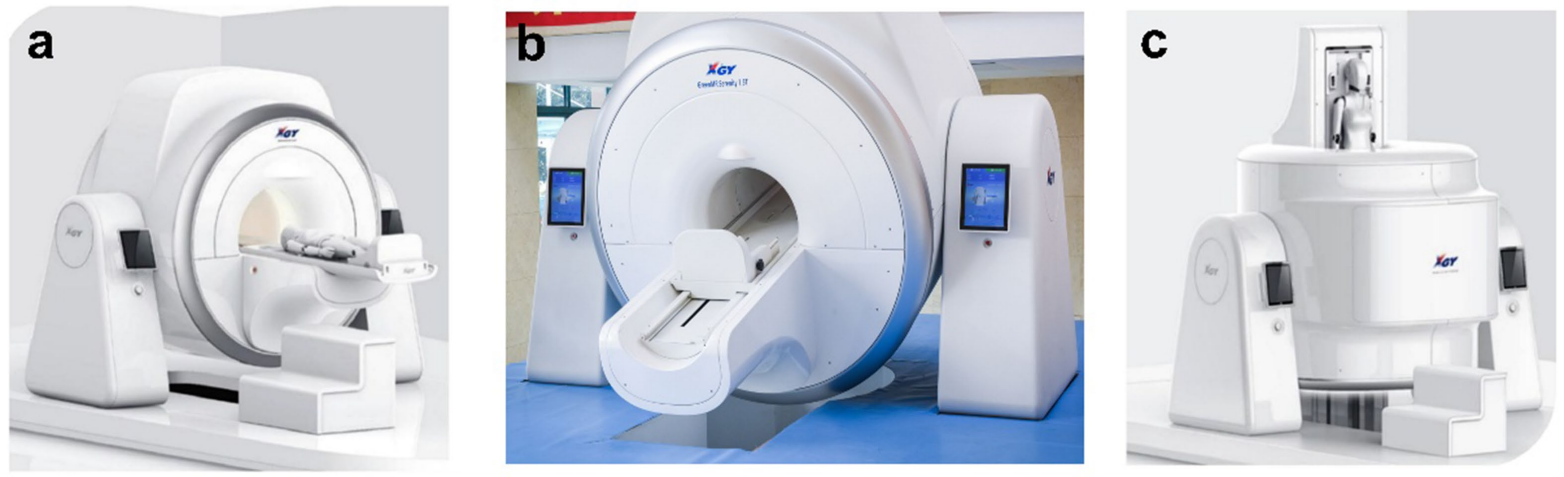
Design and configuration of the 1.5T superconductive cryogen-free spin MRI system: (a) at 0° angle for supine scanning, (b) at 45° angle for an oblique view, and (c) at 90° angle for upright scanning.
